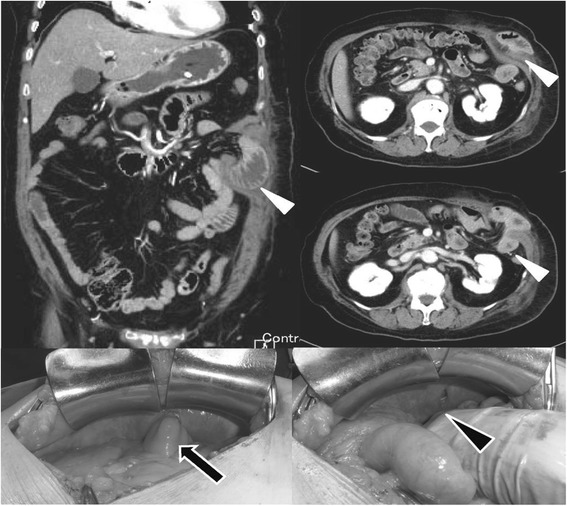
Small bowel loops identified in the upper abdomen. Upper panel: a CT scan of the abdomen identifying the ventral hernial defect with accompanying prolapse of the bowel (shown as white arrowhead). Bottom panel: perioperative photograph showing the impacted intestine (black arrow) and the defect in the muscle layer (black arrowhead).
